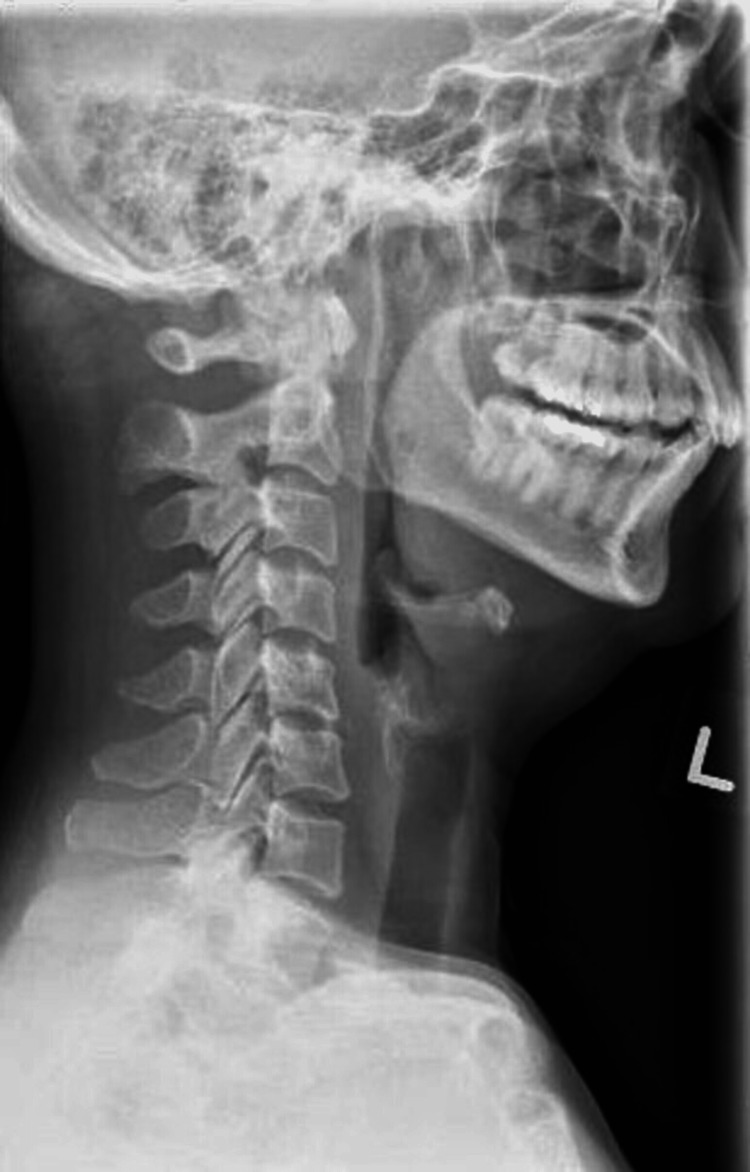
Lateral neck plain radiograph
